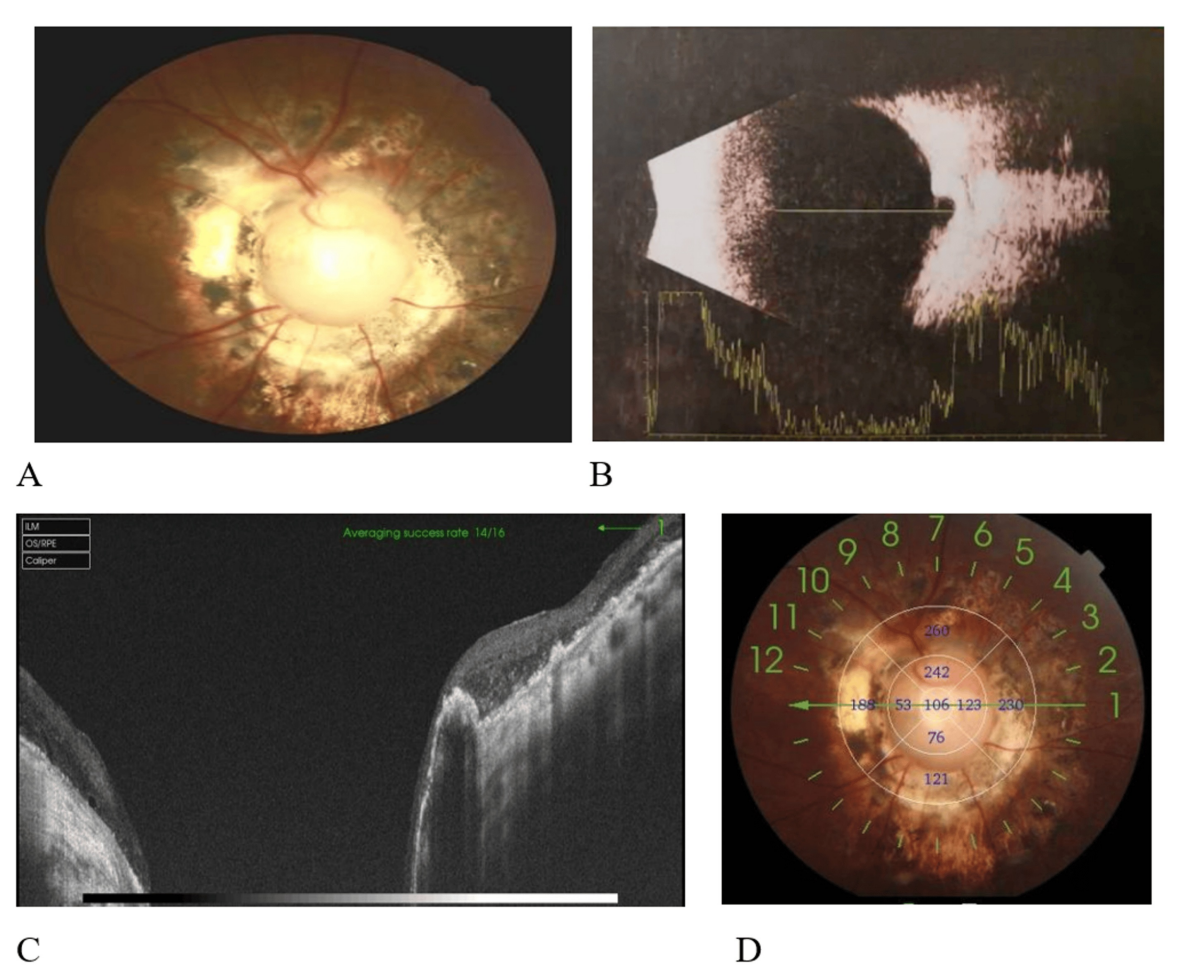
Postoperative ocular examination six months after surgery (A) At six months post-surgery, fundus photography of the right eye revealed normal retinal morphology. Pigment hyperplasia and atrophic thinning of the retina around the optic disc were visible, with a best corrected visual acuity of 2/100. (B) B-mode ultrasound examination of the right eye demonstrated retinal reattachment, with visible concave changes in the optic disc. (C and D) Optical coherence tomography (OCT) scans of the optic disc showed retinal reattachment.
